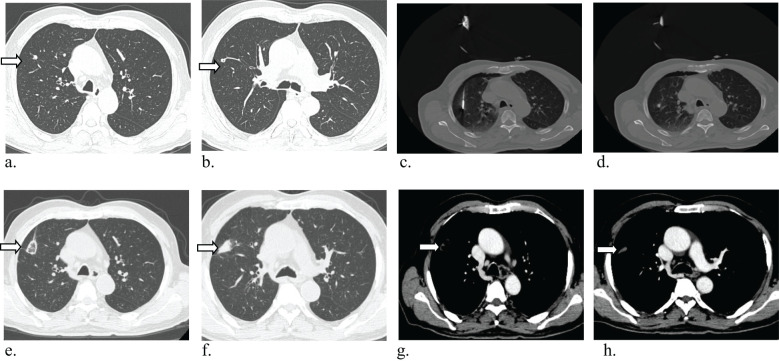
Axial CT images in 81-year-old man with multiple lung metastases from colorectal cancer, and the CRC resected 5 months ago. (a, b) Baseline image shows Baseline imaging shows two solid lung nodules in the right upper lobes(arrow), radiographic examination indicated lung metastases. (c, d) Microwave ablation (MWA) treatment for two lesions. (e–h) CT image obtained one month after MWA shows that the tumor was well covered by ablative zone (arrow).
